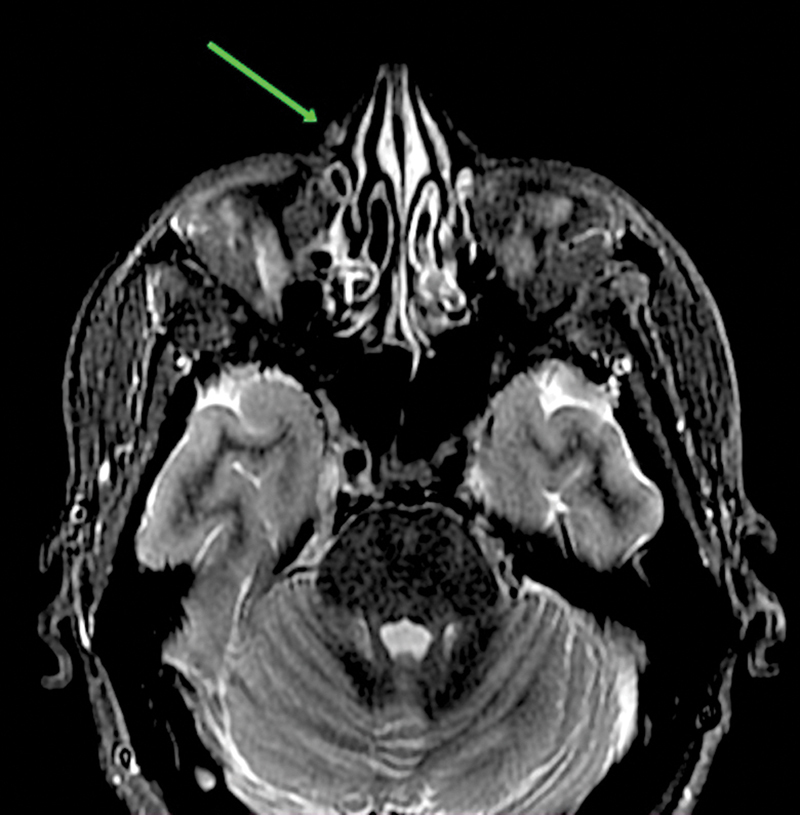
MRI showing altered signal intensity lesion and contrast-enhanced nodule in the right lateral nasal wall.
